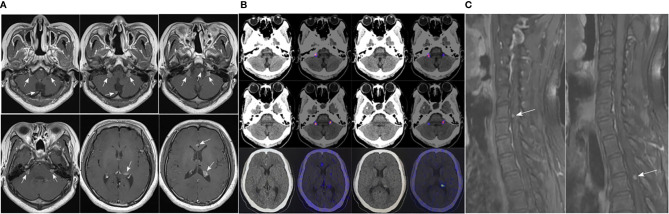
(A) Pituitary enhanced MRI in October 2020 indicated: multiple enhanced nodules appeared in bilateral auditory nerve area, pons, medulla oblongata and near cerebral falx; (B) 68Ga DOTA-TATE PET-CT in March 2021 showed: no abnormal increase in 68Ga DOTA-TATE uptake was seen in the low-density shadow in the sella turcica; 68Ga DOTA-TATE uptakes in multiple soft tissue nodules in the brain (obvious in the bilateral jugular foramen area, corpus callosum knee, left ventricular triangle, etc.) increased; (C) Before the patient underwent whole-brain radiotherapy, a spinal cord enhanced MRI was completed, which revealed abnormal enhanced nodules in the sixth cervical vertebrae and fourth thoracic vertebral plane of the spinal cord.
